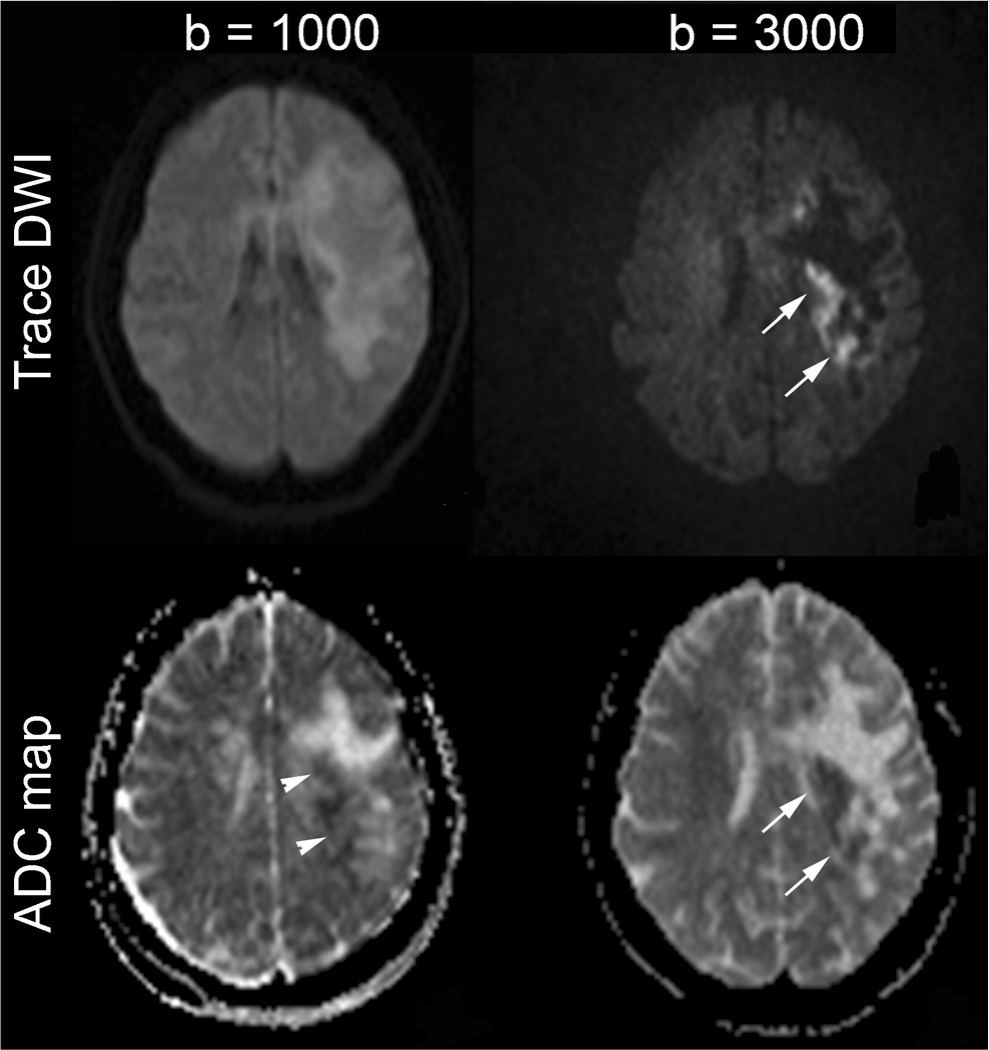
At b1000 the hyperintense progressive multifocal leukoencephalopathy (PML) rim is a heterogeneous region that corresponds to intermediate apparent diffusion coefficient (ADC) values on average, although some spots of low ADC values within the rim are observed (*arrowheads*). However the rim on b3000 diffusion-weighted imaging (DWI) contains a higher proportion of low ADC values (*arrows*), suggesting that the more stringent delineation of the lesion rim on b3000 results in exclusion of some areas with increased diffusion
